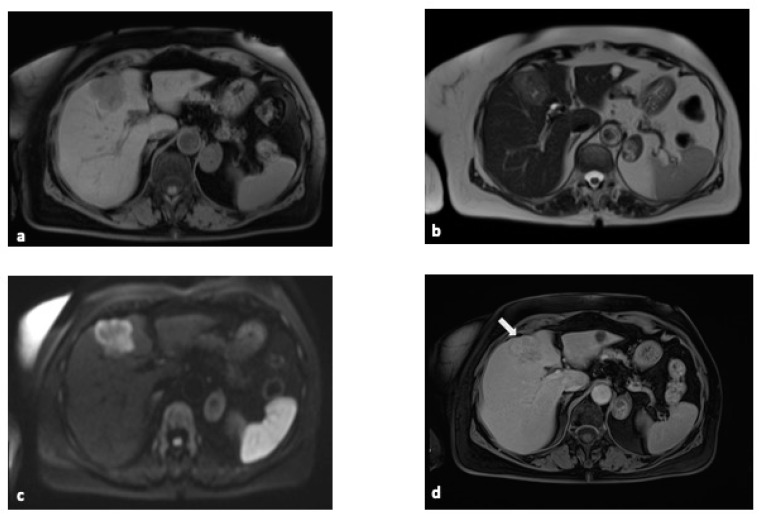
MRI features of iCCA. MRI features in a 77-year-old female with a diagnosis of intrahepatic cholangiocarcinoma: (a) hypointense on the T1-weighted sequence; (b) hyperintense on the T2-weighted sequence; (c) target appearance in DWI images: peripheral hyperintensity and central hypointensity; and (d) progressive enhancement in DCE-MRI images. A retraction of the hepatic capsule can also be observed (arrow). Abbreviations: MRI, magnetic resonance imaging; DWI, diffusion-weighted imaging; DCE-MRI, dynamic contrast sequences.
